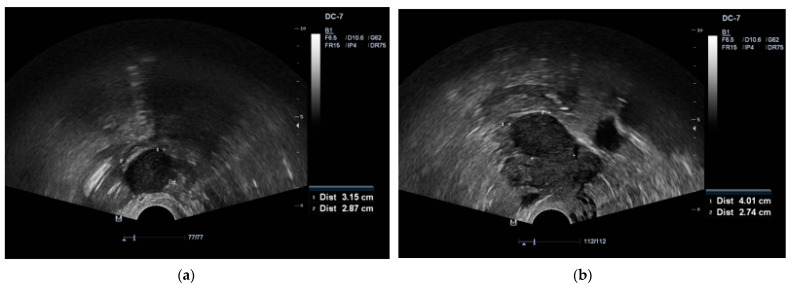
(a) Transvaginal ultrasound of a 3.1 × 2.8 cm lesion in the right ovary. (b) Transvaginal ultrasound of a 4.0 × 2.7 cm lesion in the left ovary.
